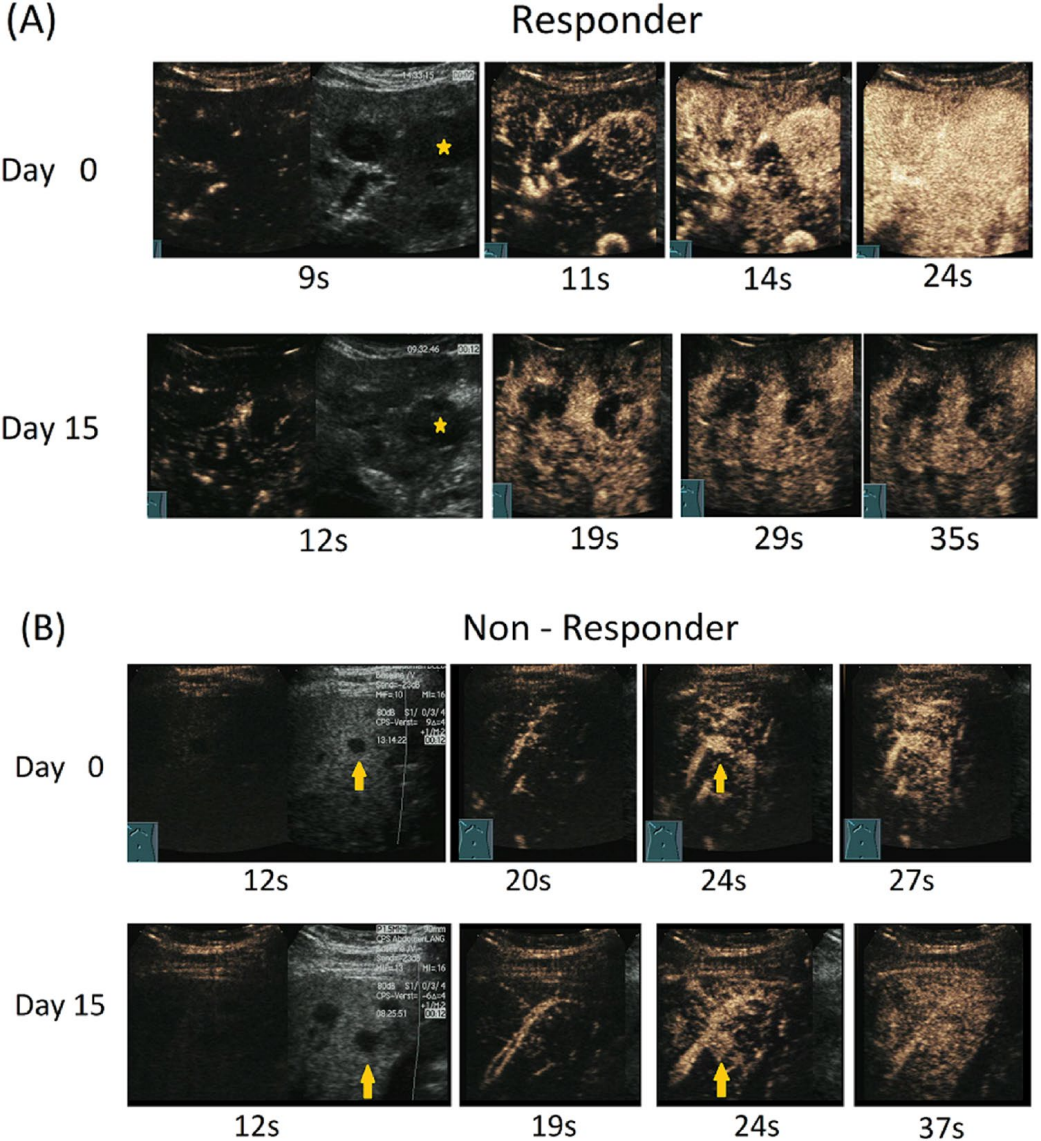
Contrast-enhanced ultrasound examinations: The first image showing the dual window mode with the lesion in grey scale ultrasonography and at the beginning of contrast agent wash-in. Image series of two patients after initiation of sorafenib therapy at baseline (d0) and after 2 weeks of treatment (d15). a Patient with response to sorafenib treatment. A decline of signal intensity within the tumor and the occurrence of avascular areas (remaining black) in the lesion (*) are visible. The tumor size is unchanged. b Patient without response to treatment. The lesion (↑) remains well vascularized throughout the arterial wash-in phase. Again, the lesion size is constant
